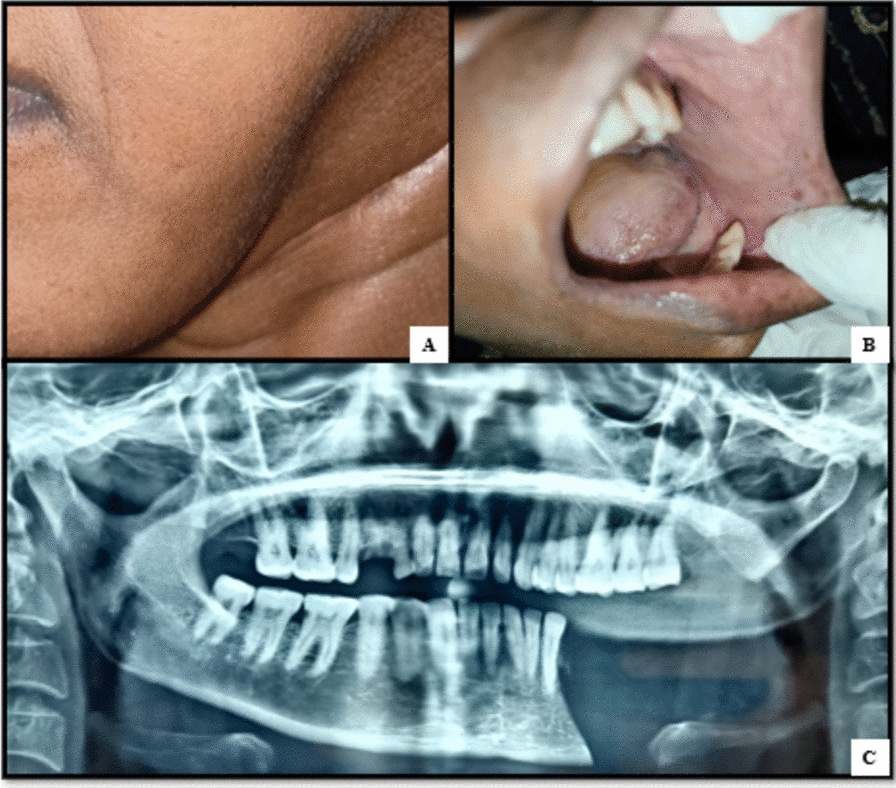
A Postoperative photograph showing facial disfigurement on left side of face, B intraoral postoperative photograph showing missing left mandibular segment distal to 34, and C orthopantomogram showing surgical defect involving body and ramus of mandible on left side
